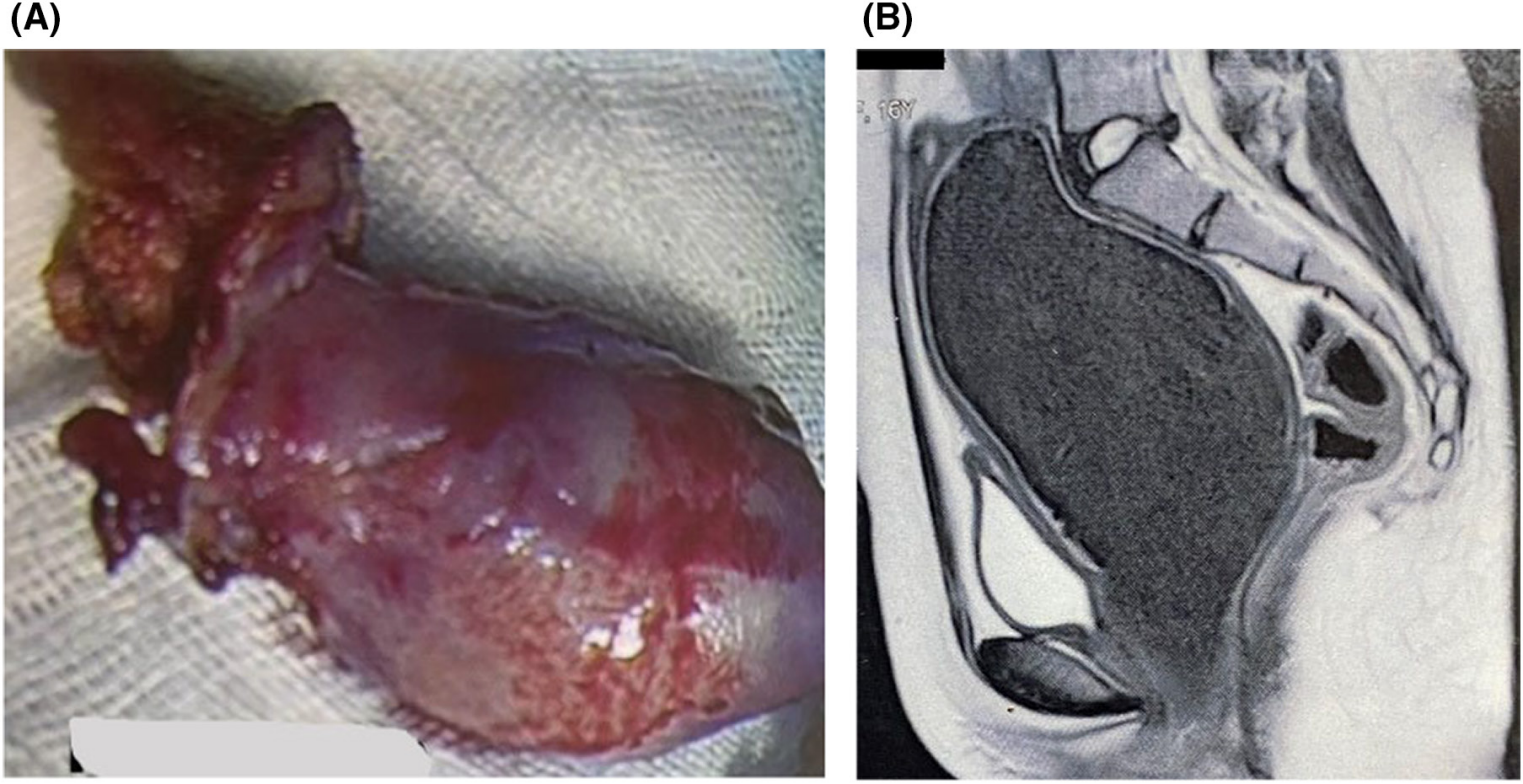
Patient's imaging findings and surgical interventions 4 years before presenting to us. (A) Rudimentary uterine horn resection; (B) hematocolpos revealed by magnetic resonance imaging (MRI).
